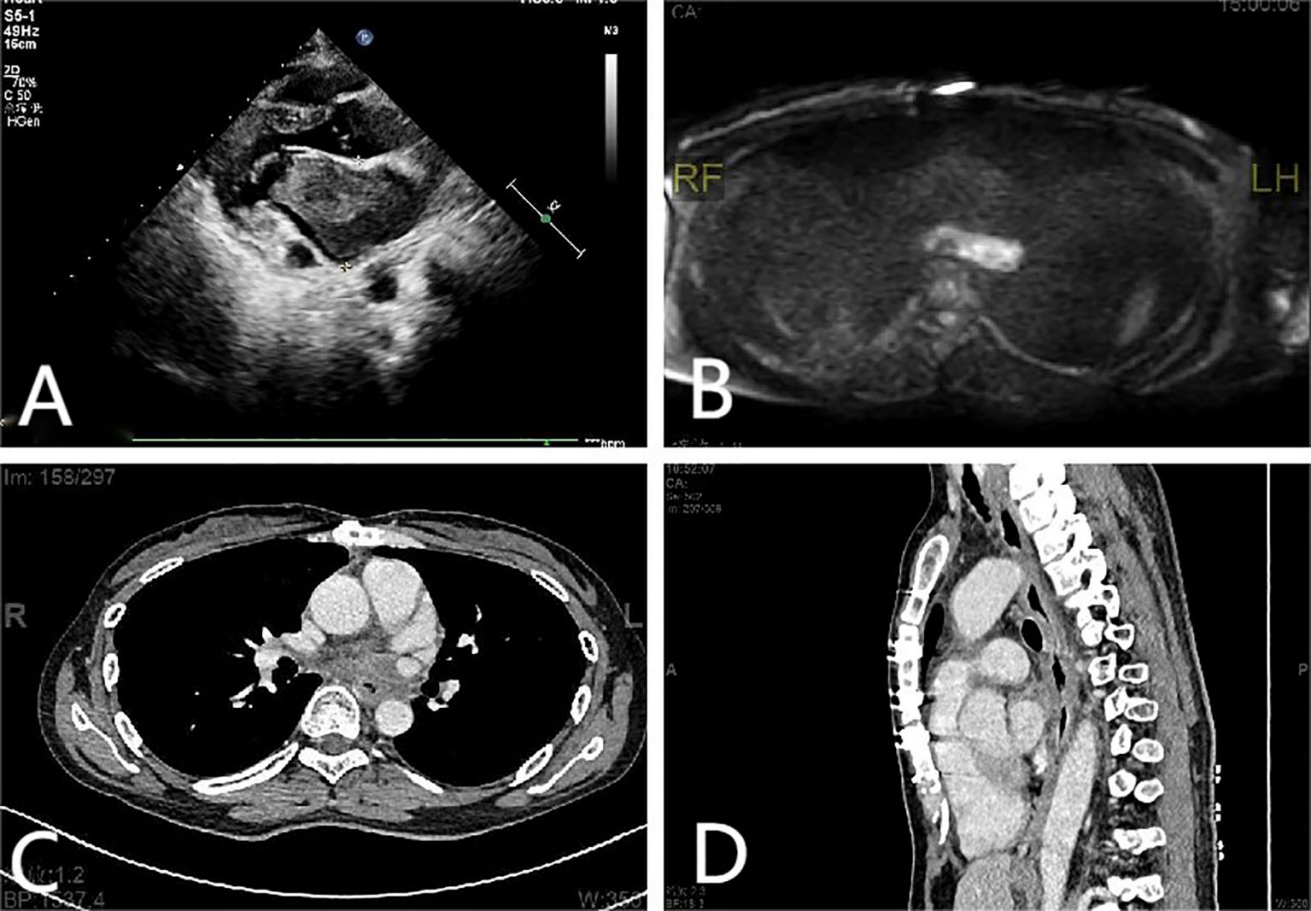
(A) Echocardiographic findings: A mass measuring approximately 69 mm × 41 mm was identified in the anterior wall of the left atrium. (B) Cardiac MRI scan findings: abnormal signals in the posterior wall of the left atrium. (C, D) Mediastinal CT scan findings: the posterior wall of the left atrium and the posterior esophagus were thickened. The gap between the esophagus and the left atrium disappeared, and tumor cell infiltration of the esophagus was considered.
